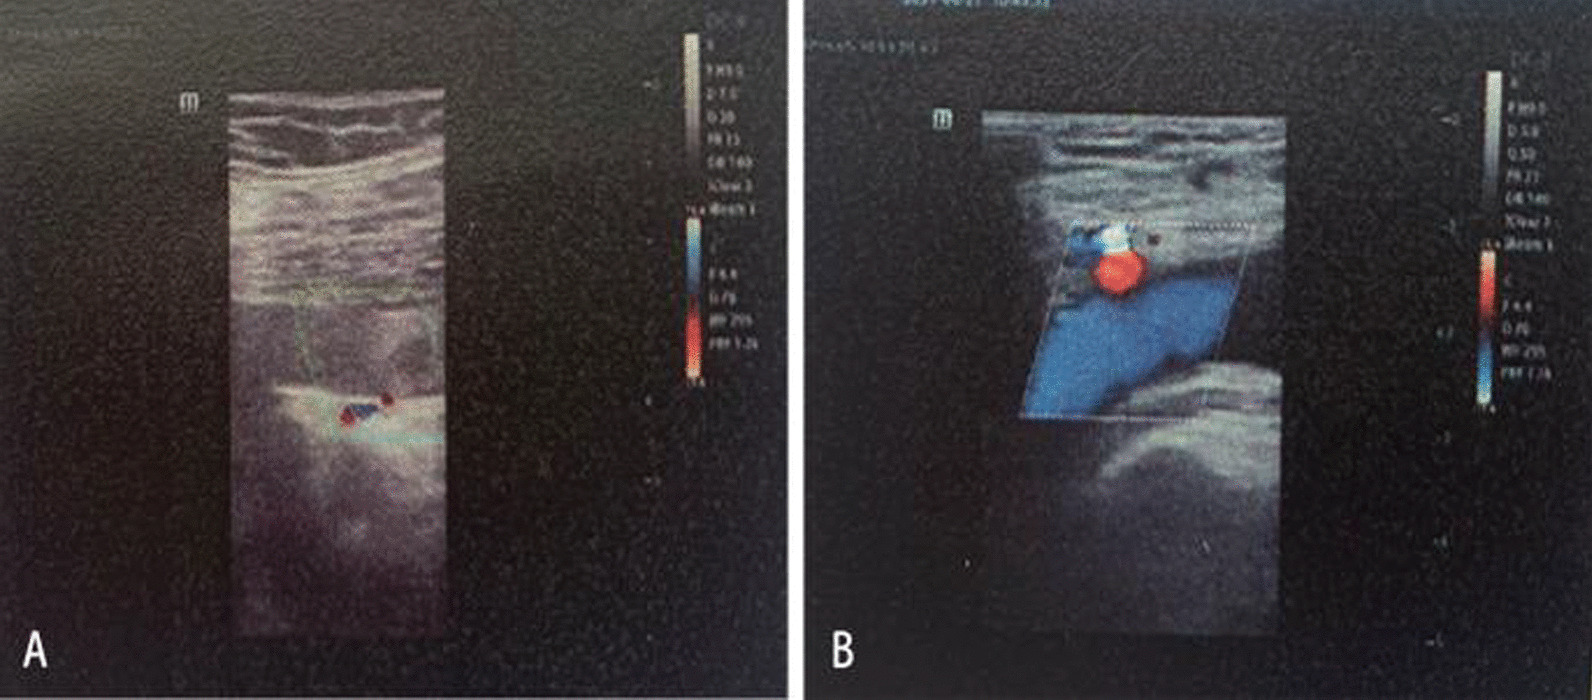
A, B Primary ultrasonography indicated the possibility of deep venous thrombosis (DVT) of the right lower extremity
